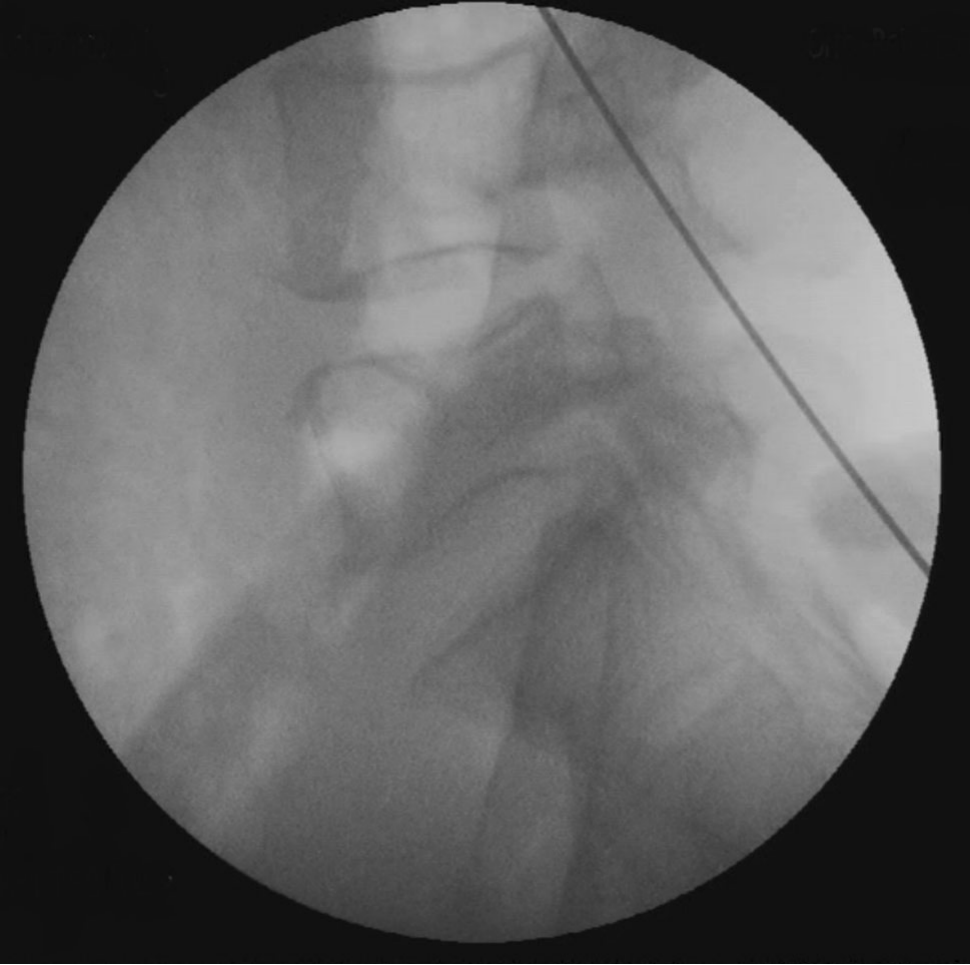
Wire placed over the pars interarticularis on lateral view X-ray
